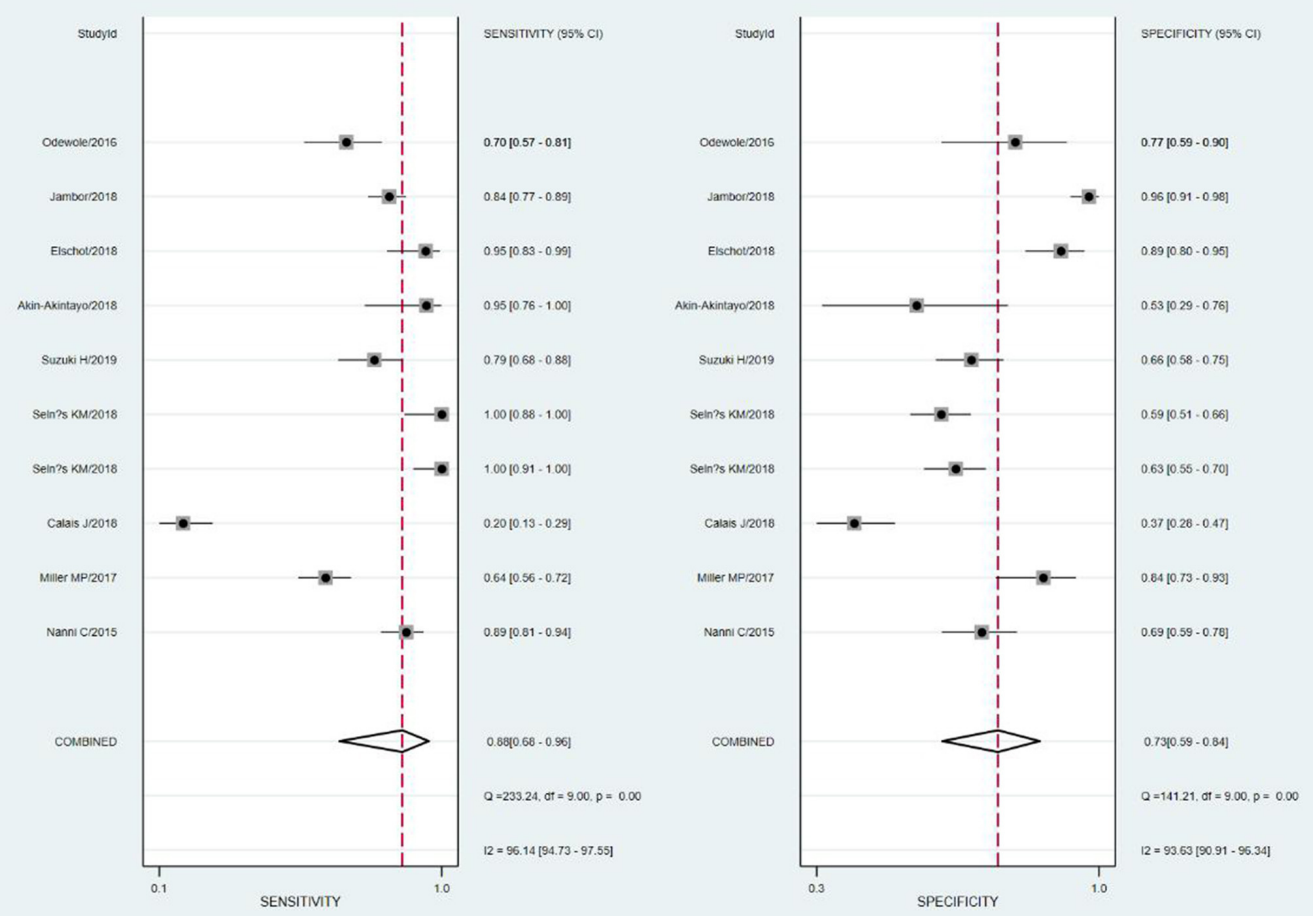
Forest map of 18F-fluciclovine PET/CT for diagnosis of prostate cancer.
